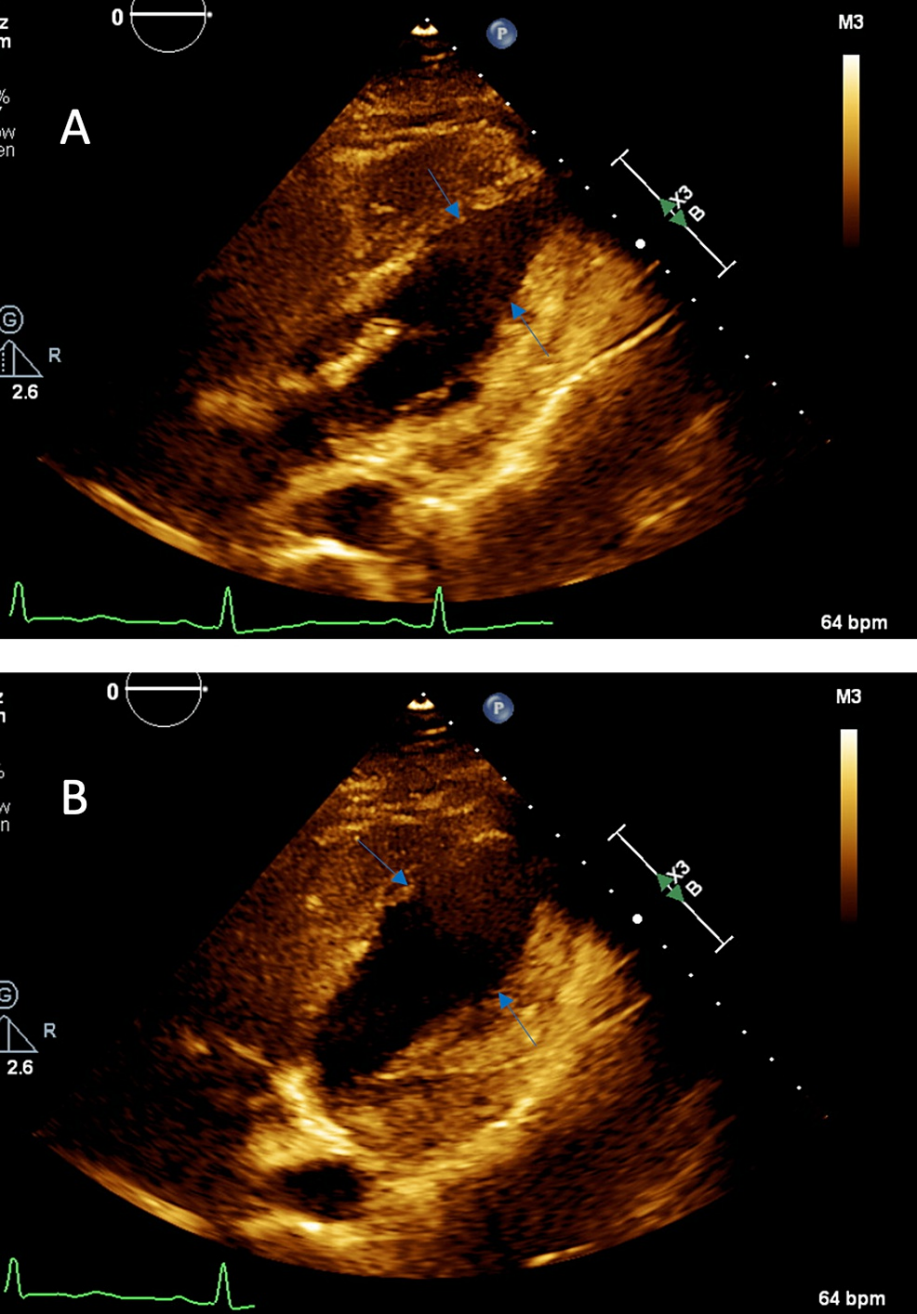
Two-month echocardiography follow-up in (A) end-diastolic phase and (B) end-systolic phase. Arrows point to resolution of ventricular wall abnormalities.
